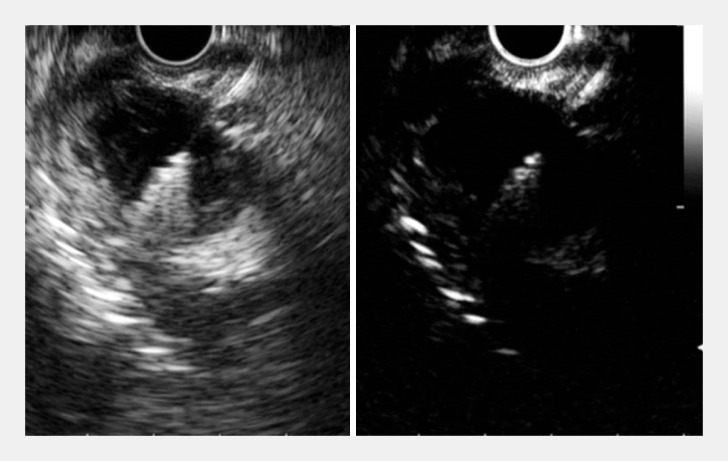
Contrast-enhanced endoscopic ultrasound images showing fine-needle biopsy (FNB), with a 22-gauge FNB needle, being successfully and accurately performed for the tumor.
